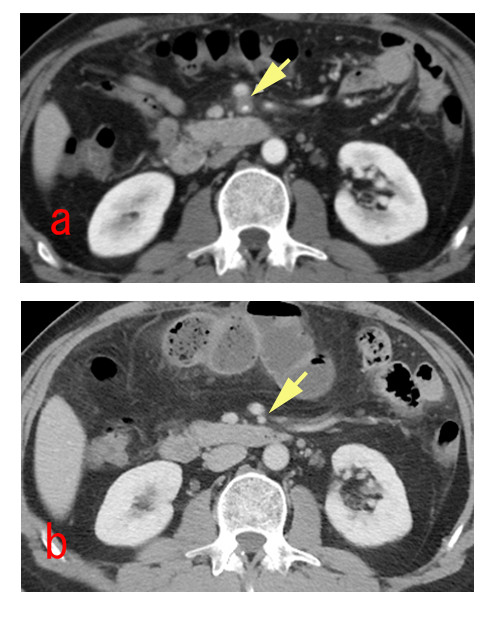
Sakamoto's type IV dissection of the SMA. (a) preoperative abdominal enhanced CT scan show isolated dissection of the SMA in which the false lumen was thrombosed without ulcer like projection(ULP). (b) postoperative 1 year abdominal enhanced CT scan show a thrombosed false lumen completely resolved with narrow true lumen.
